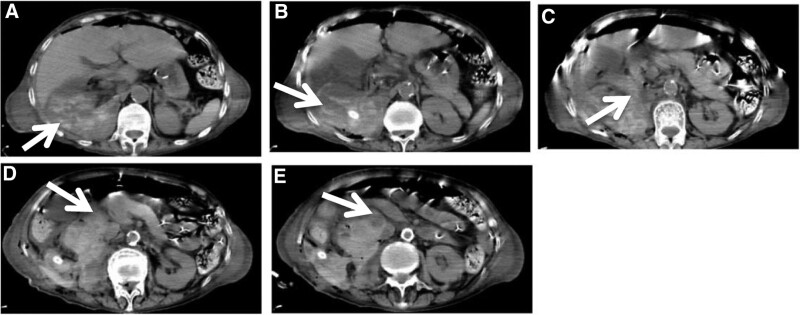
Postoperative computed tomography (CT) images of retroperitoneal hematoma after radical nephrectomy and compression of the adjacent duodenum. CT images showed the location and size of the retroperitoneal hematoma after radical nephrectomy. The retroperitoneal hematoma compressed the adjacent structures, including the duodenal wall. The arrows indicated the boundary of the hematoma (in all panels) and the relationship between the hematoma and the duodenum in panels C, D, and E.
